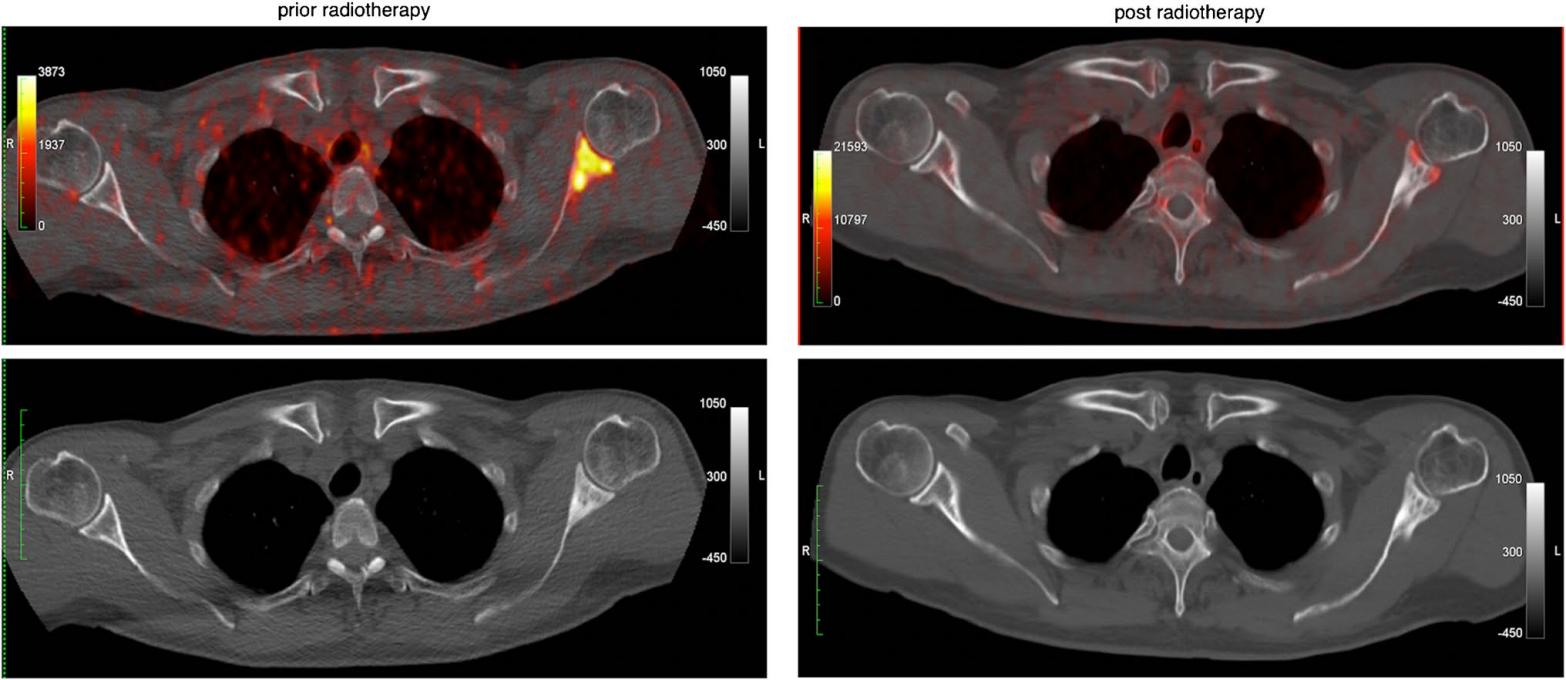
Follow-up of a patient after radiation of a shoulder metastasis in transversal section planes. The upper row shows fused PET-CT images, the lower row the native CT images. The left column shows the initial presentation with a PSA of 0.57 ng/mL after prostatectomy. This revealed a clear bone metastasis in the left scapula (SUVmax late 8.98, SUVmean late 3.09). In the native CT image at the bottom left, the bone metastasis is sclerotic. The right column shows the [^68^ Ga]Ga-PSMA-11 PET-CT images 2 years later. The shoulder metastasis has since been irradiated and no longer shows increased activity in the upper fused PET-CT image. Only a residual spot-like uptake can be seen. Osteoblastic changes are still visible on the native CT image in the lower right image. The patient came for follow-up with a rising PSA (2.49 ng/mL), and during the same examination, a local recurrence was detected in the [^68^ Ga]Ga-PSMA-11 PET-CT, which is not shown here
